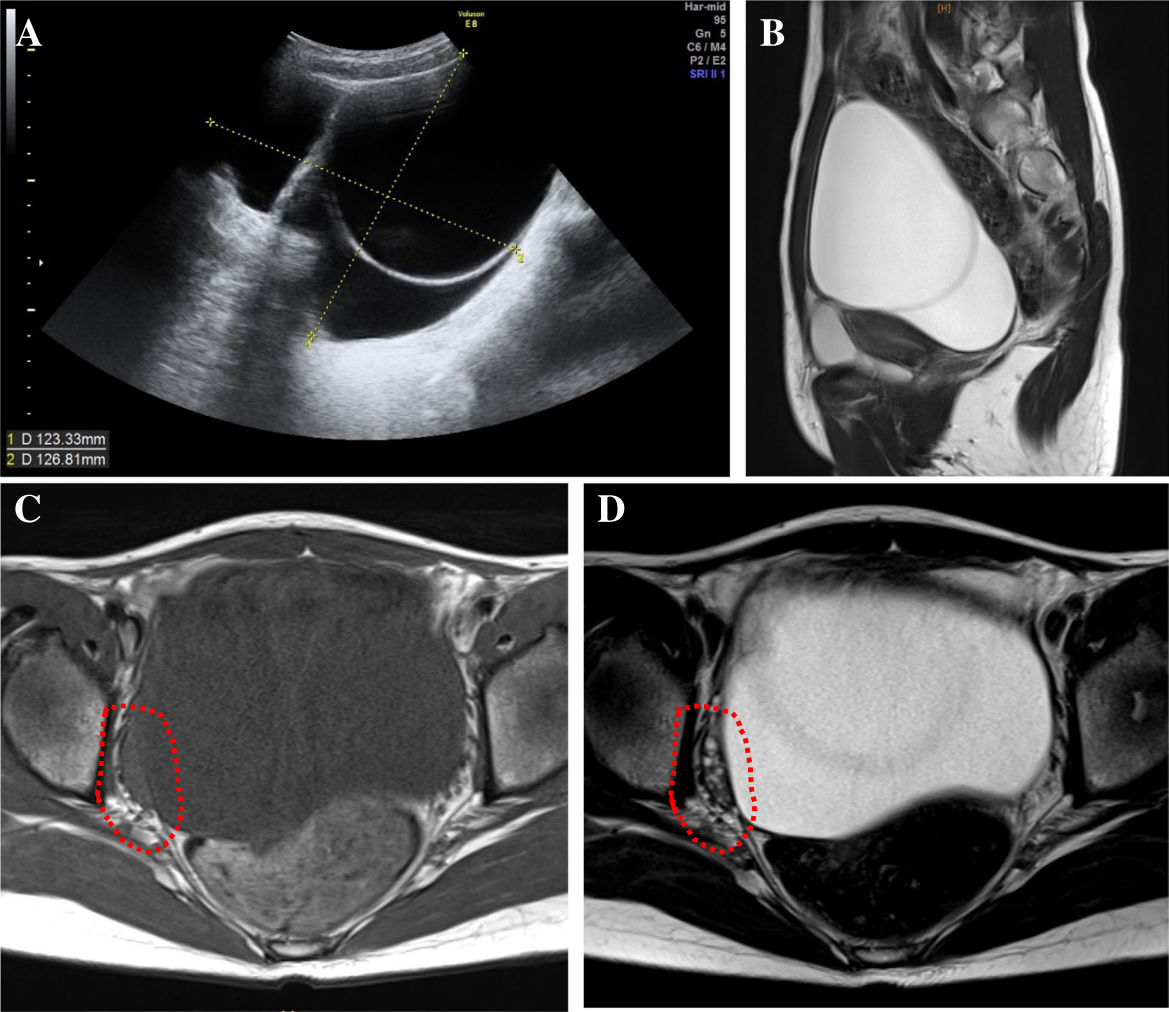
A Transabdominal ultrasonography showed multilocular cystic mass over 12 cm in diameter in pelvis. The wall of the cyst was thin and contents of each cyst were aechoic. B MRI T2 weighted sagittal image; multilocular cystic mass without any solid part occupied in pelvis. C and D MRI T1 (C) and T2 (D) weighted transverse image may indicated serous fluid contents of the cysts. Dotted circles indicate right ovary, which is polycystic appearance
